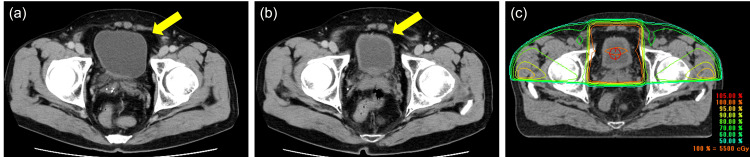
Case managed by bladder sparing therapy with avelumab and radiotherapy (Case 4) Computed tomography (CT) scan showed the primary bladder tumor (yellow arrow). (a) Pre-NAC after TURBT, (b) post-NAC, and (c) image of 3D-CRT along with isodose lines. 3D-CRT, three-dimensional conformal radiation therapy. TURBT, transurethral resection of bladder tumor; NAC, neoadjuvant chemotherapy; 3D-CRT, three-dimensional conformal radiation therapy
